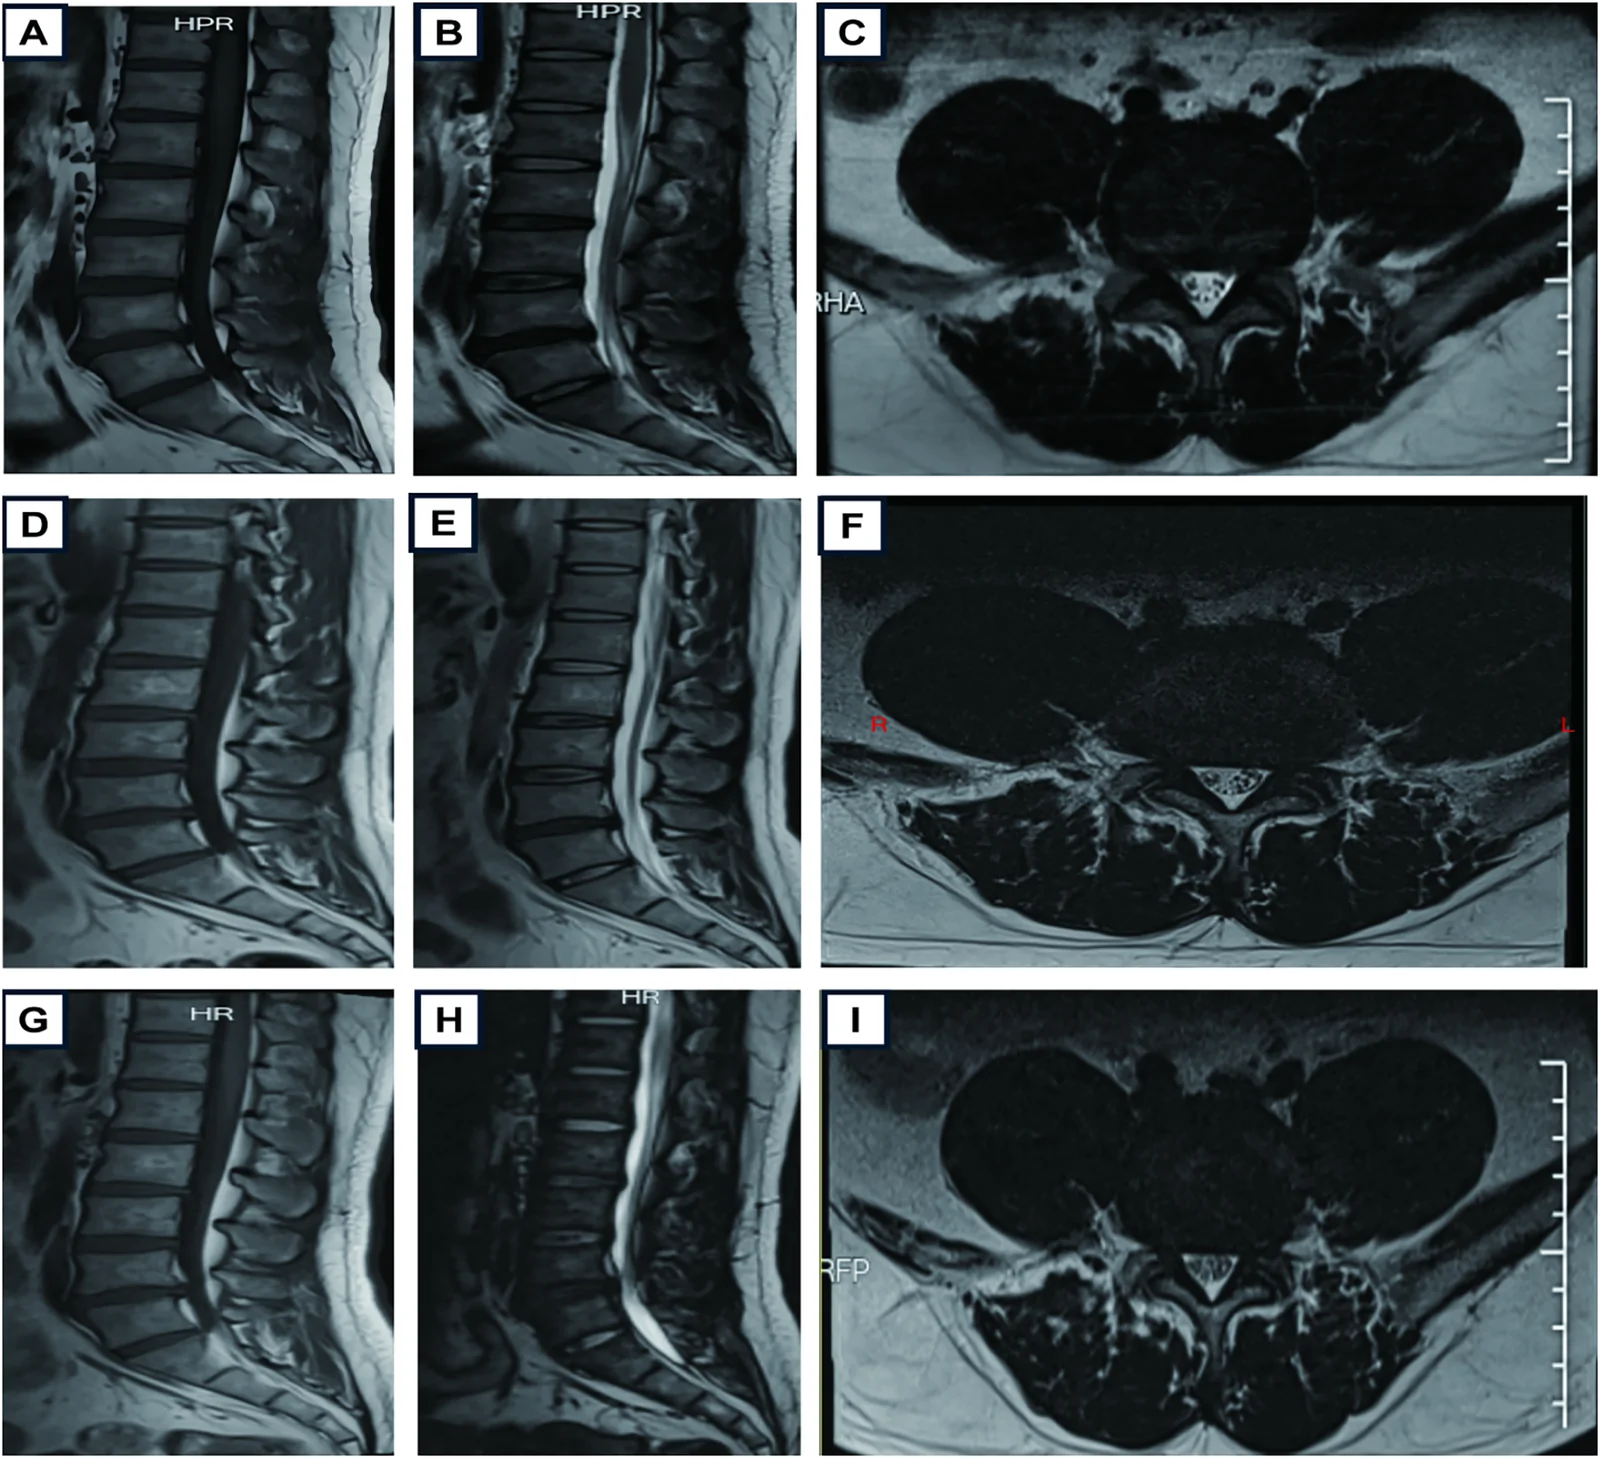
Serial follow-up lumbar MRI. (A–C) At approximately 3 months post-baseline (September 29, 2025), non-contrast MRI reveals near-complete resorption of the sequestrated nucleus pulposus at the L4/5 level. (D–F) At the 4-month follow-up (October 14, 2025), non-contrast MRI demonstrates complete resorption of the free sequestrated fragment alongside marked regression of the extruded component, leaving only a persistent residual disc bulge (herniation ratio: 17.2%; area-based resorption rate: 77.7%). (G–I) At the 9-month longitudinal follow-up (March 5, 2026; 5 months after treatment cessation), MRI shows a stable, persistent L4/5 disc bulge without evidence of recurrence or renewed nerve root compression.
